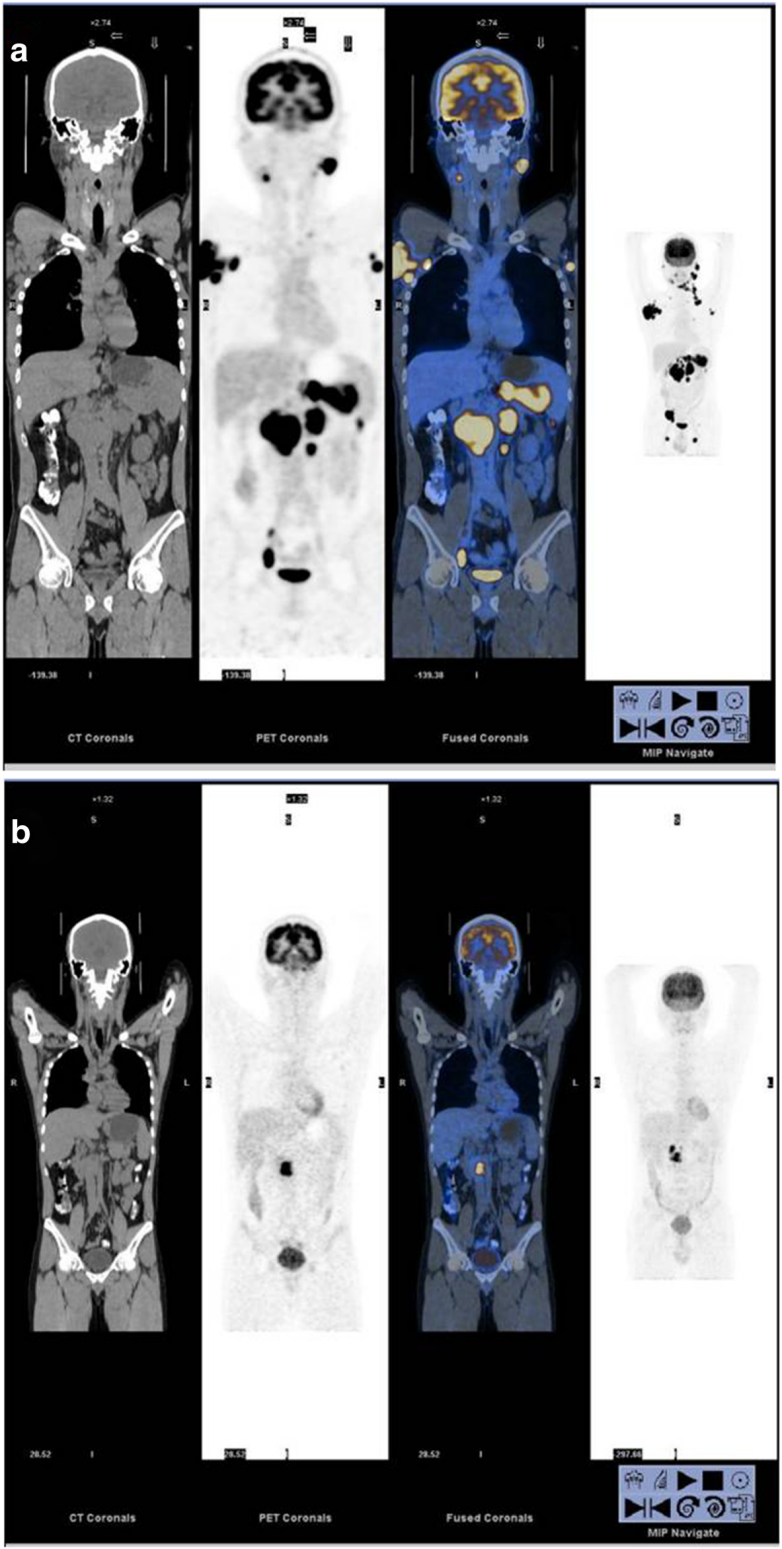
PET/CT scans of DLBCL with a score of 5 in a 45 year-old man. (a), baseline PET/CT; (b), F-PET/CT. Baseline PET/CT shows an Ann Arbor stage IV tumor, which was interpreted as positive on F-PET/CT using the QVTA criteria; the residual foci (*red arrow*) were diagnosed and scored as 5. This patient received involved-field radiotherapy after 6 cycles of chemotherapy using rituximab, cyclophosphamide, doxorubicin, vincristine, and prednisone (R-CHOP) regimen, but the disease progressed, and the patient died 31 months after treatment initiation
